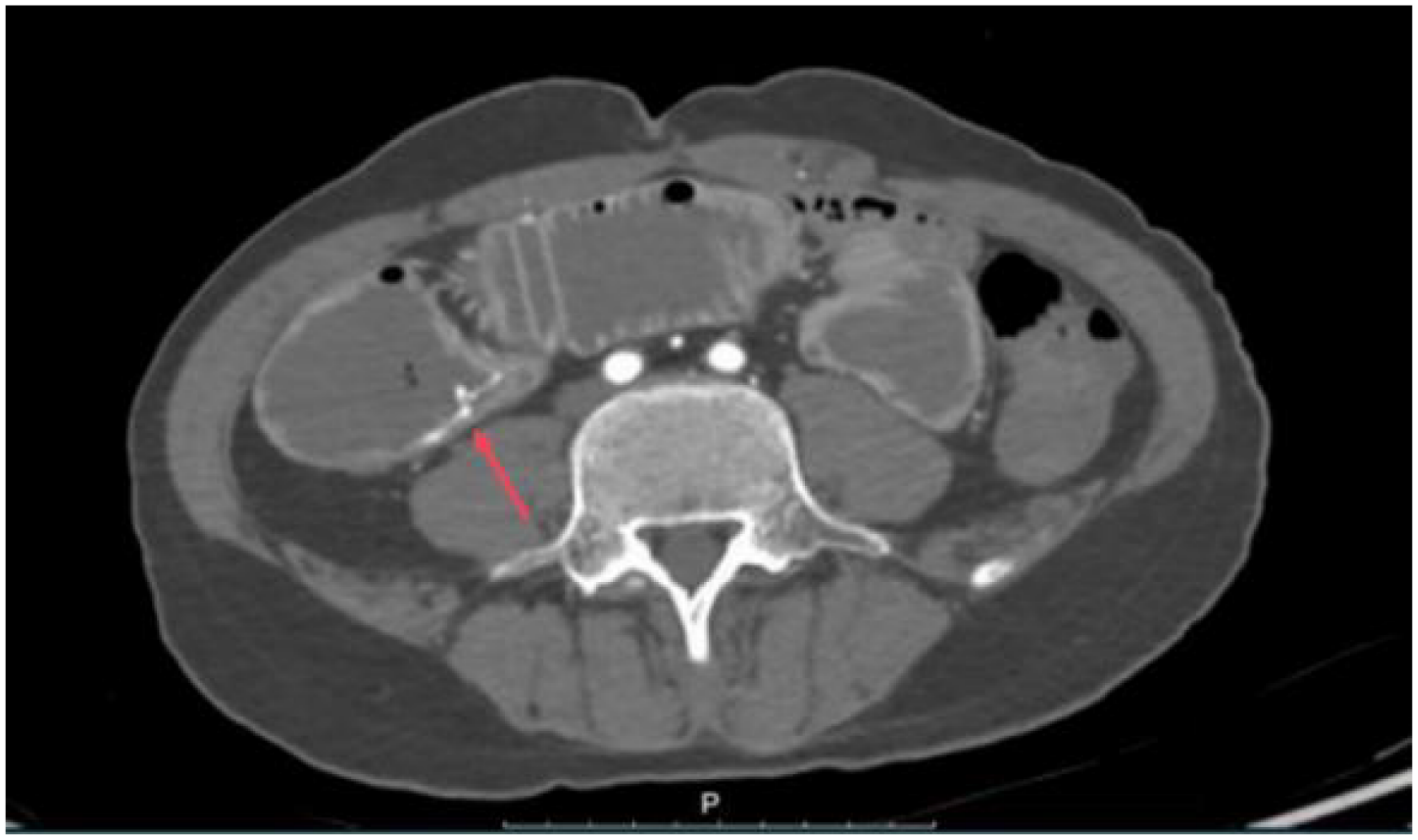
Mesenteric artery computed tomography scan revealing that one branch of the mesenteric artery had apparent extravasation of contrast material.
